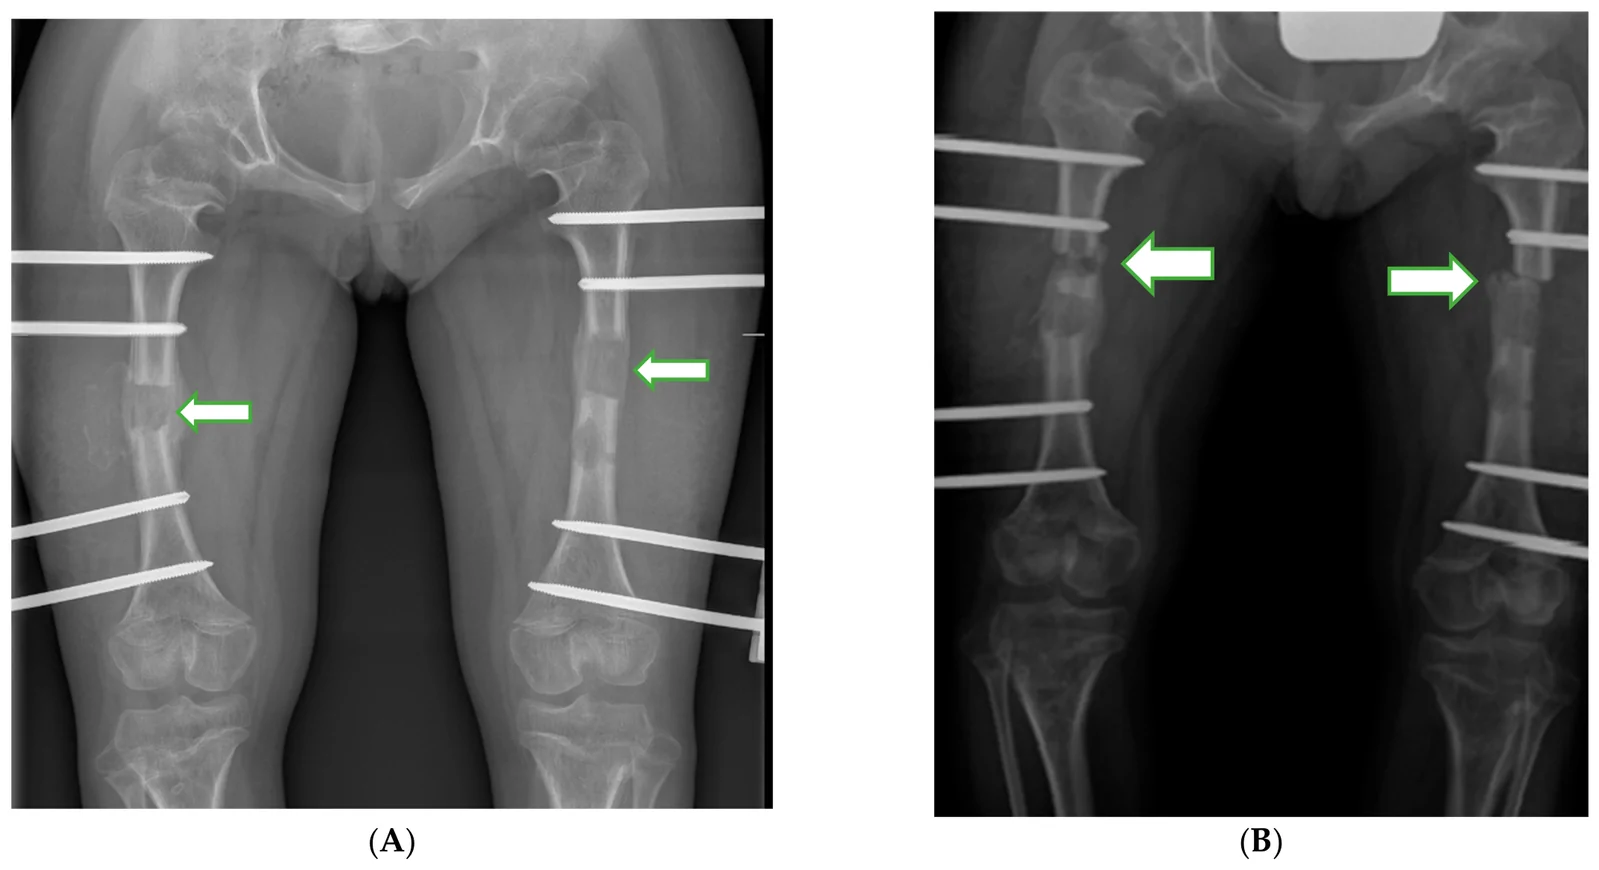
(A) X-ray showing premature consolidation of femurs bilaterally, as indicated by the white arrow. (B) X-ray showing the outcome of the calloclasis on the femurs bilaterally, as indicated by the white arrow.
